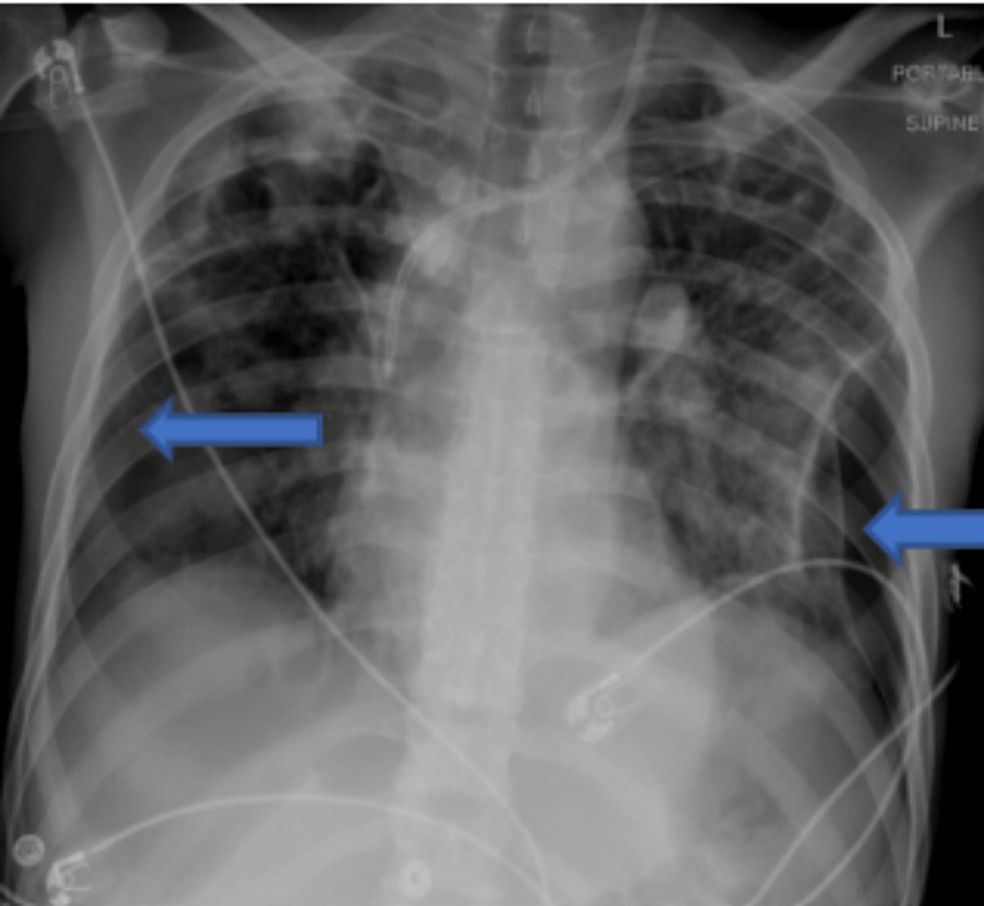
Chest X-ray showing evidence of bilateral pneumothoraces. The blue arrows indicate new left-sided loculated pneumothorax and right-sided pneumothorax.
